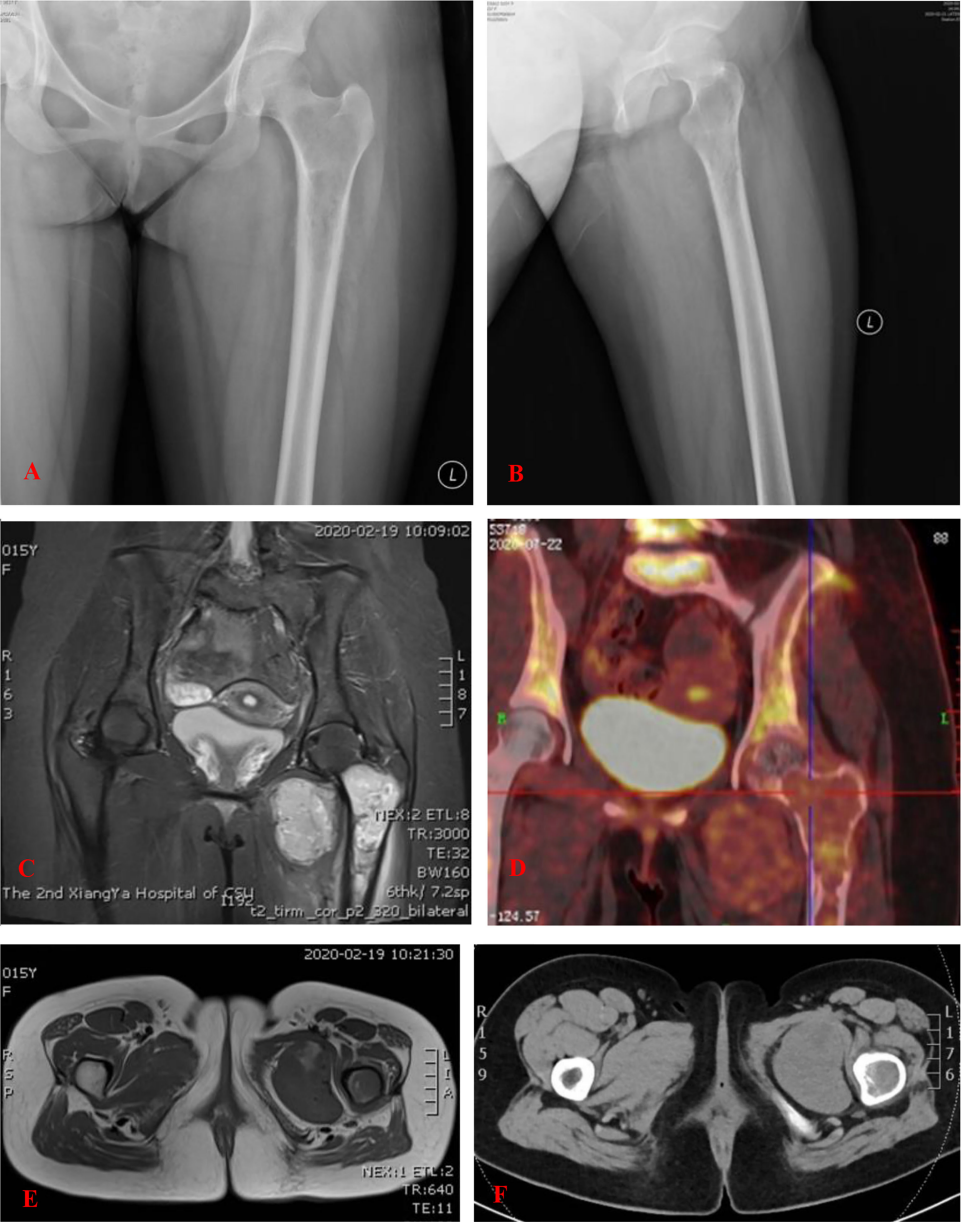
(A, B) An anteroposterior and lateral plain radiograph of the left femur. There is a comparatively well-outlined osteolytic lesion at the proximal part of the left femur. (C, E) MRI of the lesion of the proximal femur. The mass showed isointensity on T1-WI and high intensity on T2-weighted images (T2-WI). Well-defined oval-shaped heterogeneous soft tissue mass in close proximity to the medial side of the lesser trochanter. (D) PET-CT showed slight abnormal fluorodeoxyglucose (FDG) uptake in the lesion. (F) CT showed an expansile lesion at the proximal femur in the intertrochanteric region.
